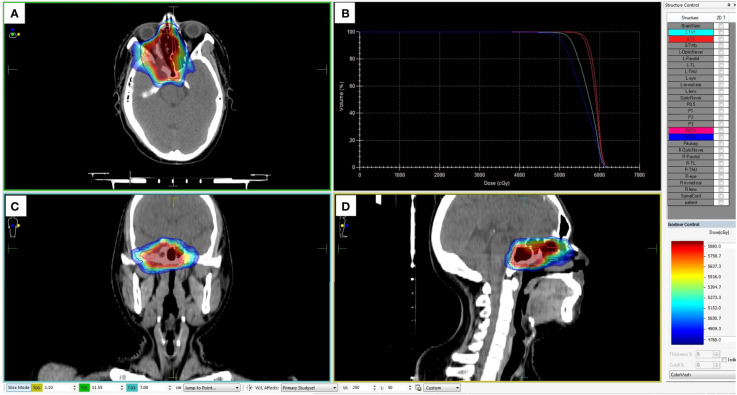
Dose planning of radiotherapy. Planned target volume (GTV: red, PGTV: pink, CTV: light blue, and PTV: blue) covered by the 95% isodose can be seen in axial (A), coronal (C), and sagittal (D) planes on pretreatment planning computed tomography. (B) The dose-volume histogram showed that 95% of the PGTV and PTV volumes irradiated with more than 5,040 cGy and 5,600 cGy.
